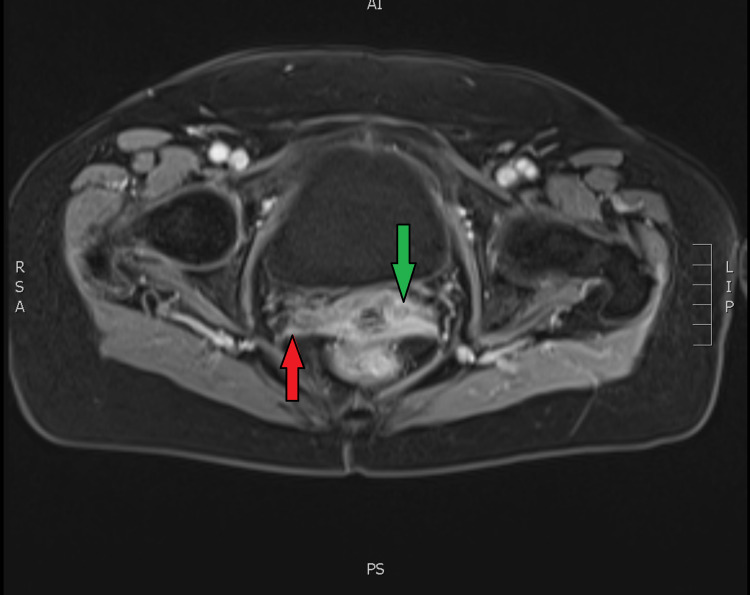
Axial view of the MRI scan Red arrow: Right parametrium; Green arrow: Axial view of the cervix
